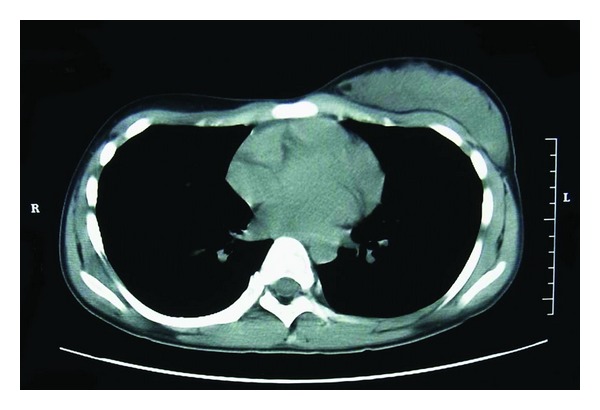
MRI: thorax and pectoralis major are symmetric. Normal mammary gland is present on the unaffected side.
